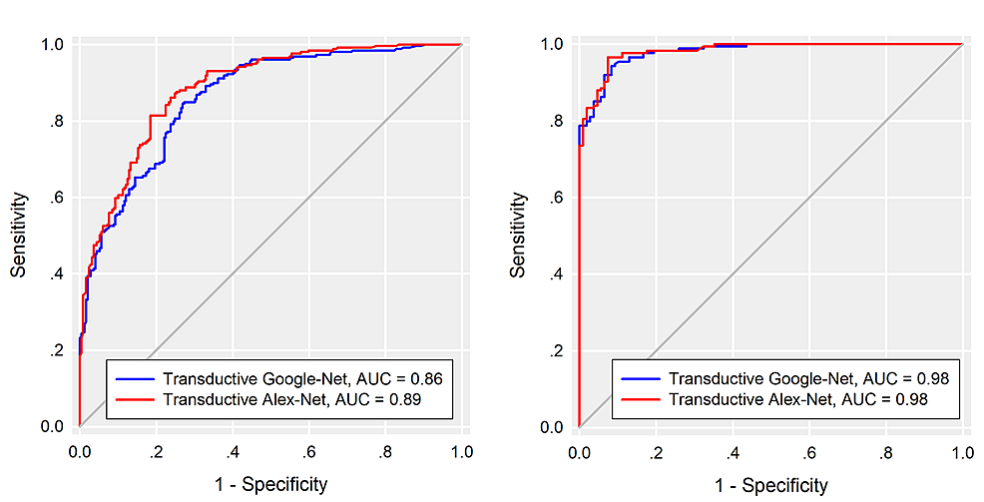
(a) AUCs of transductive TL Alex-Net and Google-Net models, T2WI protocol. (b) AUCs of transductive TL Alex-Net and Google-Net models, ADC maps. AUC - area under the curves T2WI - T2-weighted image ADC - apparent diffusion coefficient
